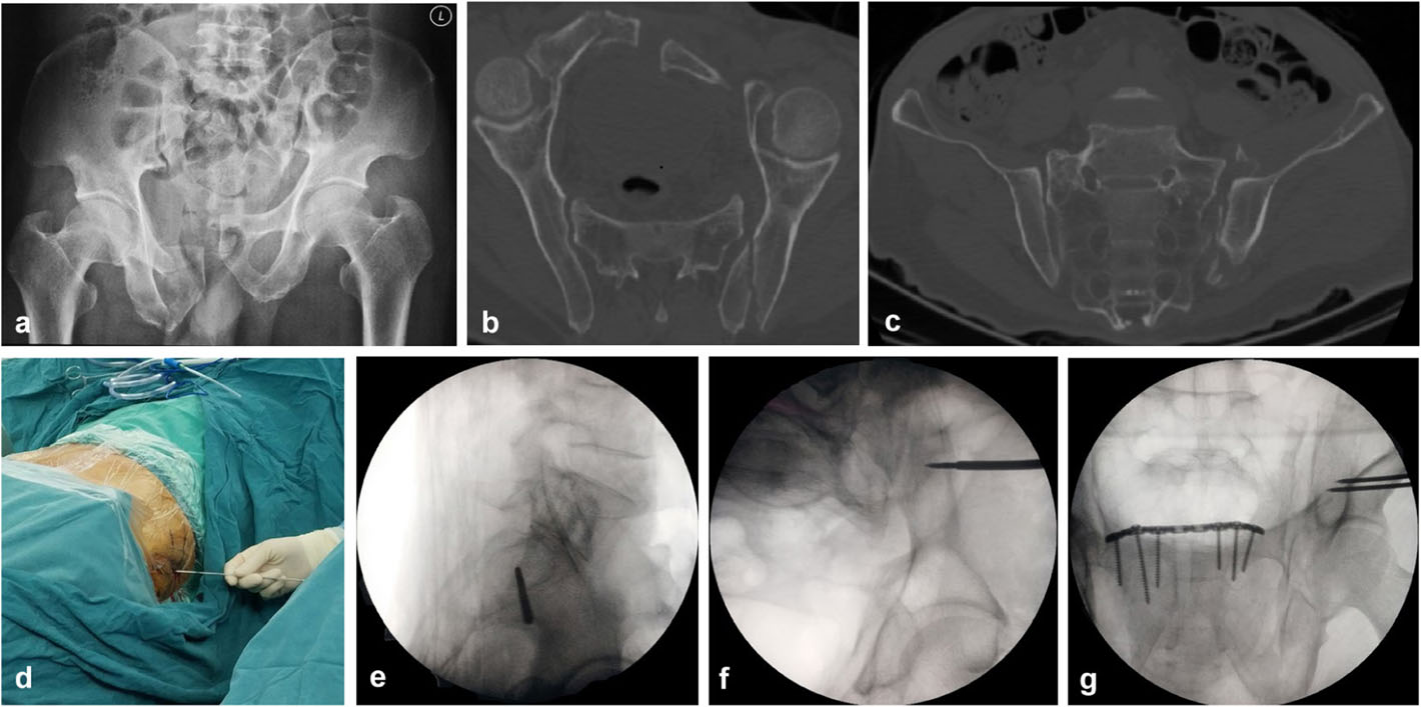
Injury anteroposterior (AP) pelvis radiograph (a). Reconstruction views of axial CT (b) and coronal CT (c) showed that there was a presence of dysmorphism at the upper sacral segment, and the second sacral segment existed a corridor for a transiliac-transsacral screw. The guidewire was inserted into the starting point on the fracture side (d) with a true lateral sacral view (e). Intraoperative fluoroscopic inlet (f) and outlet (g) views confirmed the position of guidewire was satisfactory
